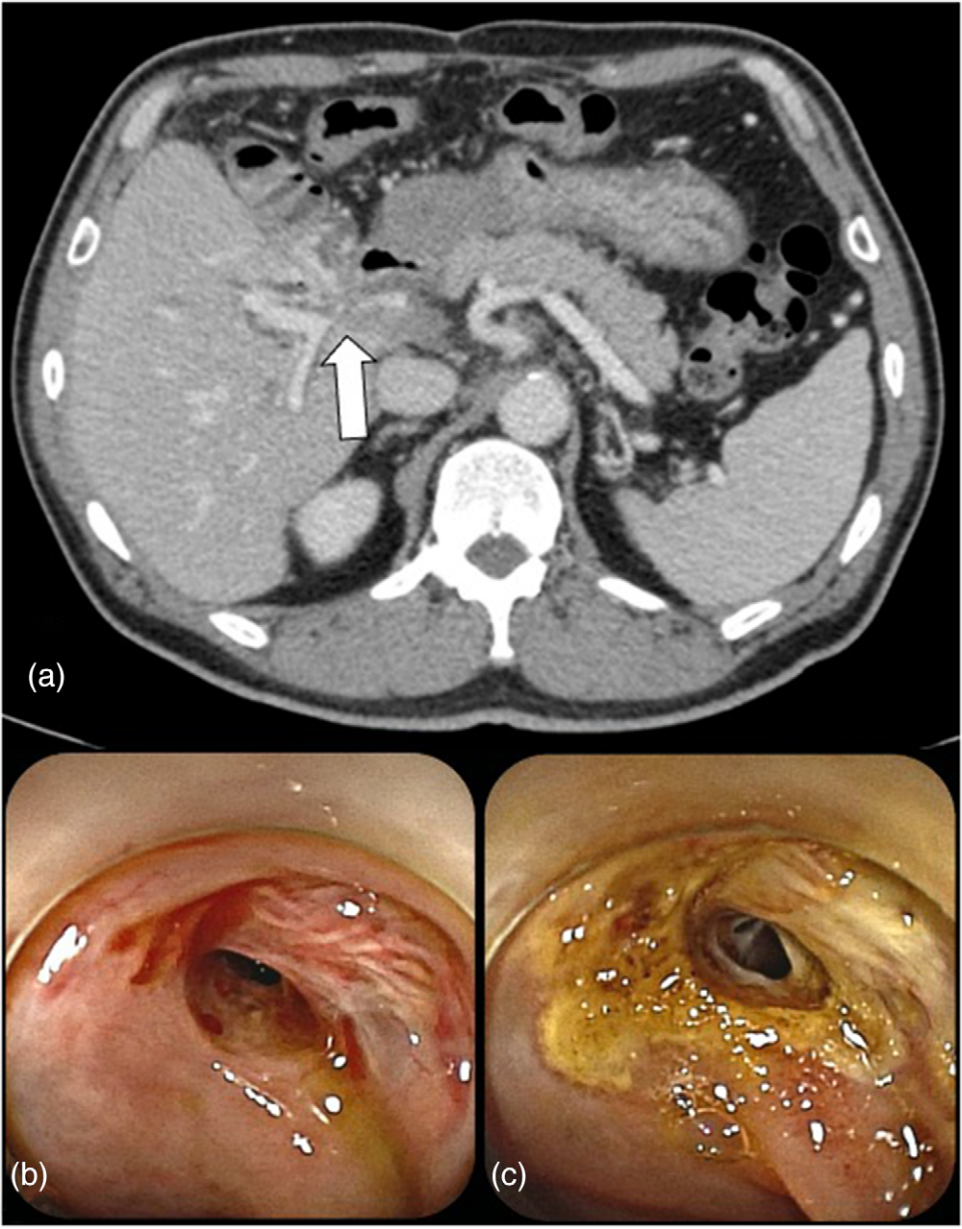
In case 3, contrast‐enhanced computed tomography showed portal vein stenosis (a). Endoscopic image shows the angiodysplasia‐like lesions around the hepaticojejunal anastomosis (b), and we employed argon plasma coagulation (c). White arrow: portal vein stenosis
